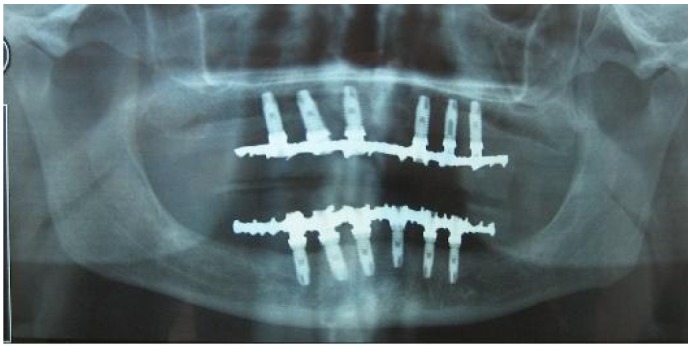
Orthopantomography of a 67-year-old patient rehabilitated with a superior hybrid prosthesis on 6 implants and a mandibular hybrid prosthesis on 6 implants.
